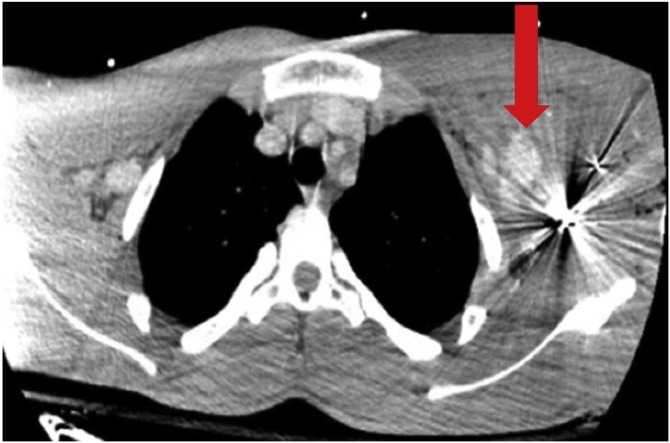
CT Angiography Chest. Gunshot wound to the left shoulder; the bullet visualized above creates artifact at the left shoulder thus obscuring visibility of the arterial injury. Nevertheless, extravasation of contrast material is evident. A large caliber bullet fragment is also noted overlying the left shoulder.
